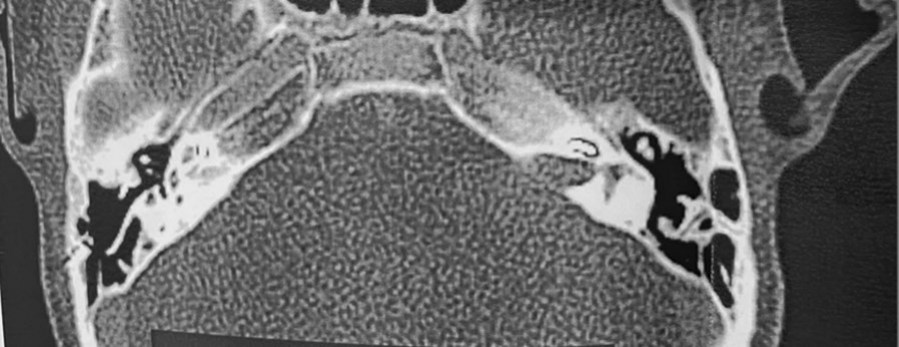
Axial contrast CT images. CI in the left basal gyrus of the cochlea and the CPA mass
